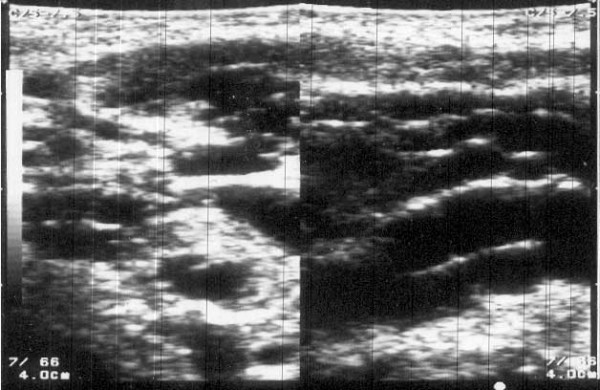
Ultrasound scan of axilla at presentation showing grossly distended lymphatics (in longitudinal and transverse axis).
